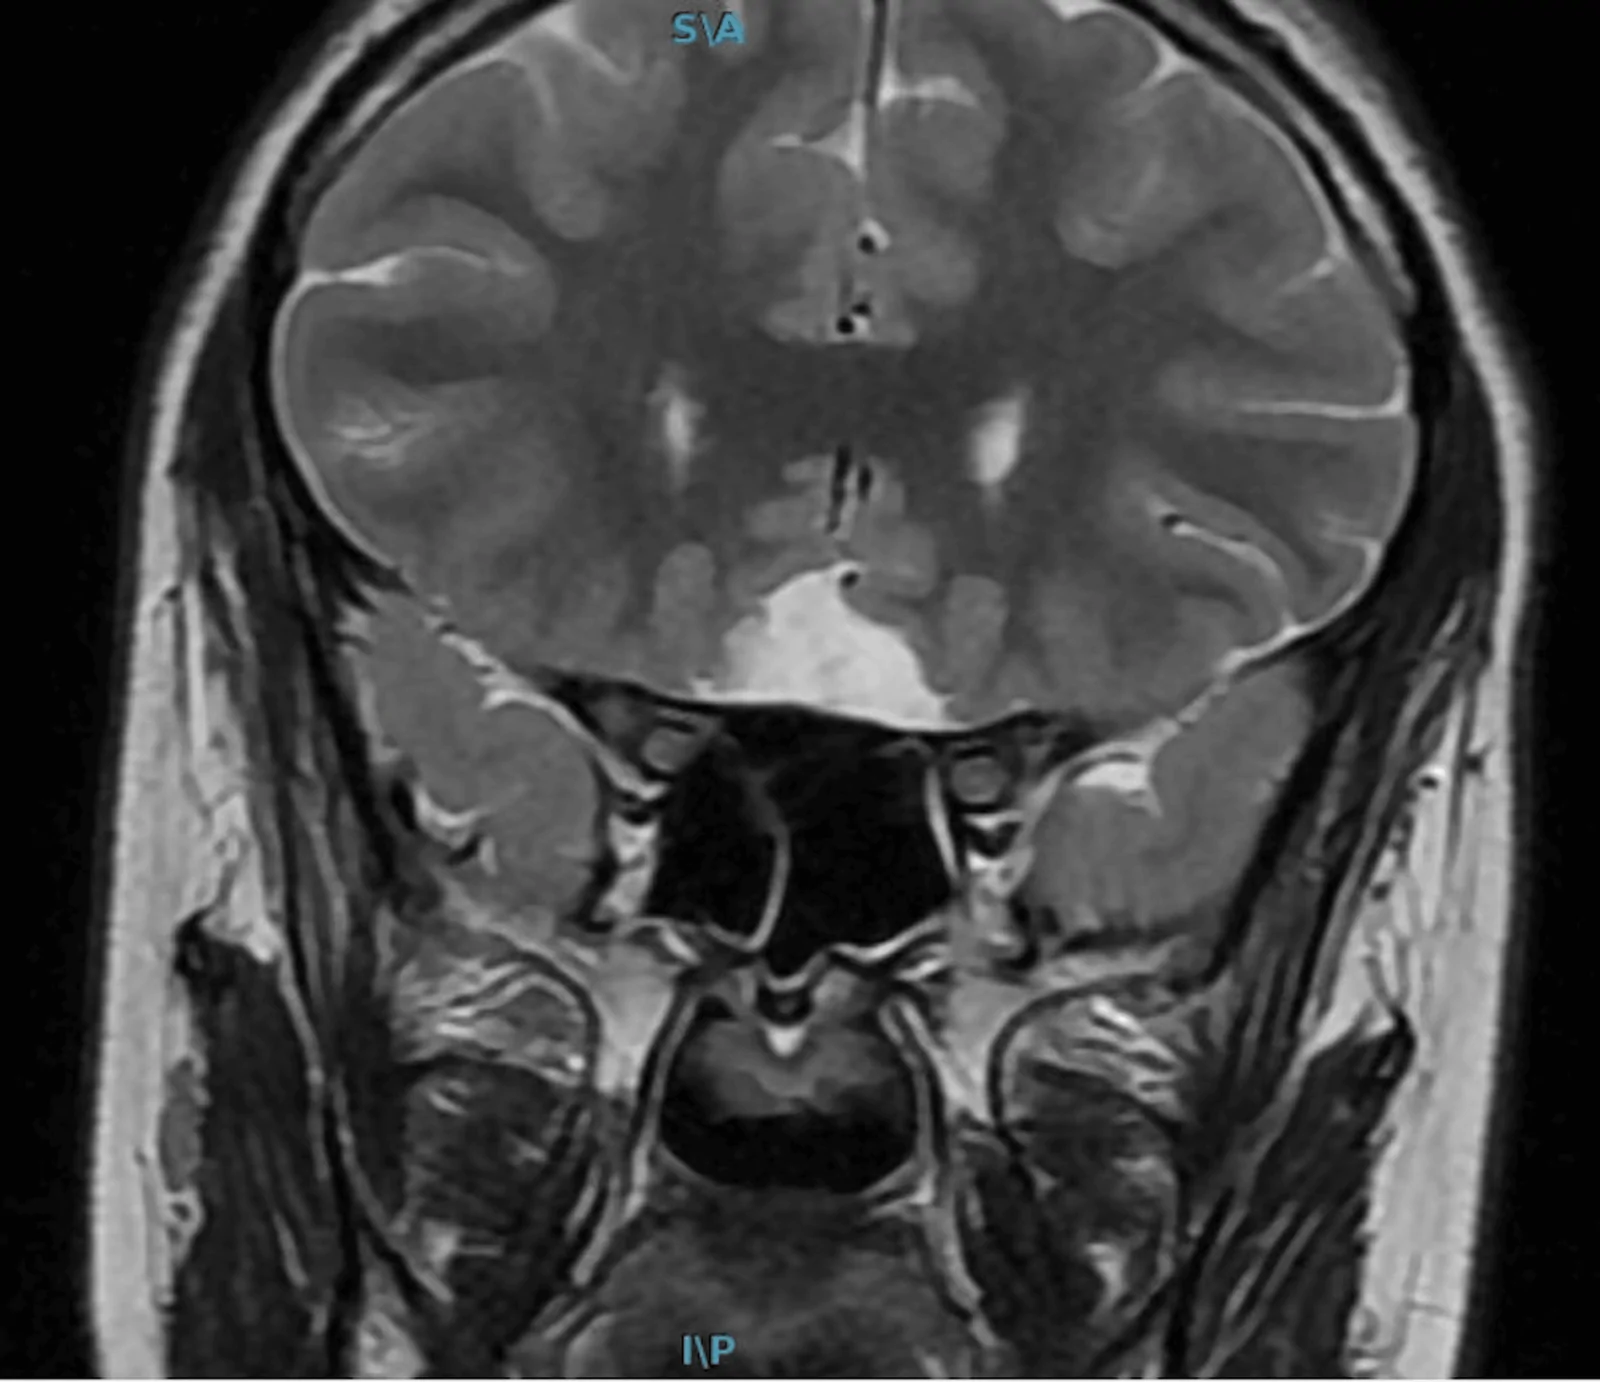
Coronal T2-weighted MRI of the sellar and suprasellar region A well-defined, hyperintense cystic lesion is seen in the sellar and suprasellar region, with mass effect on the optic chiasm. MRI: magnetic resonance imaging
